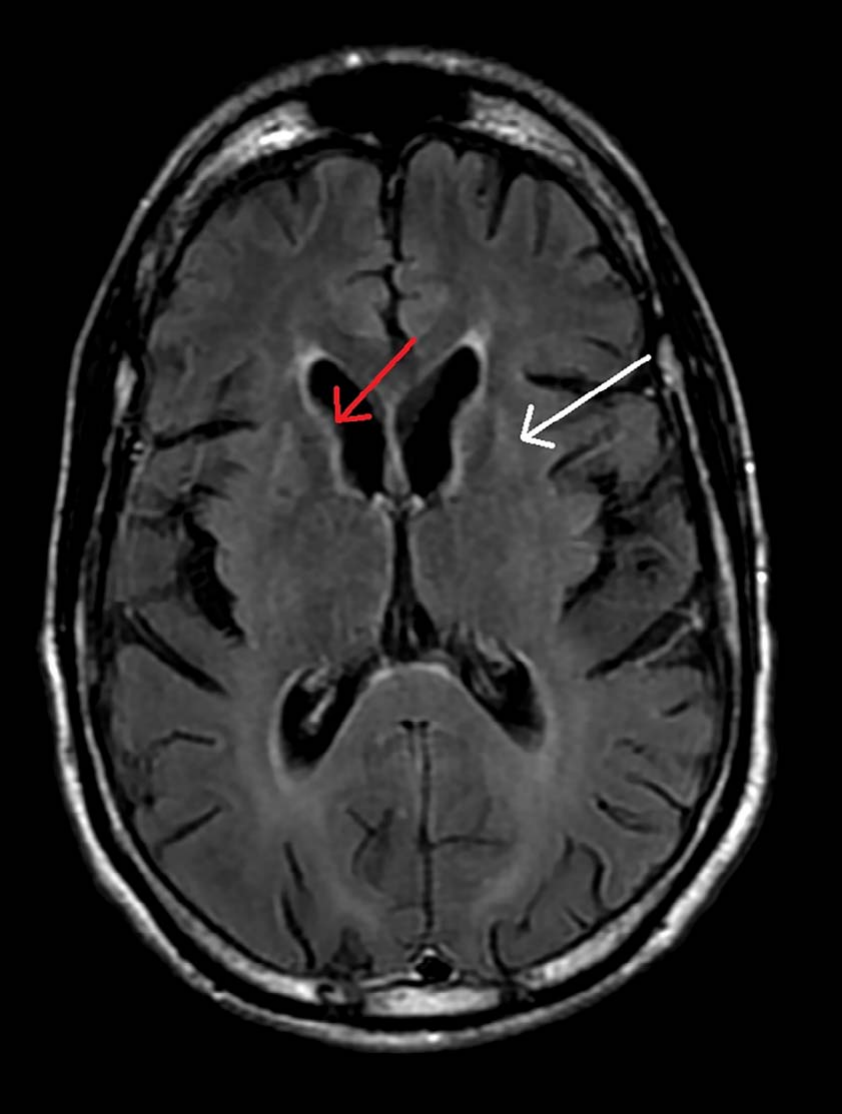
Axial T2-FLAIR (fluid-attenuated inversion recovery) magnetic resonance image of the brain of Case 2 shows severe volume loss in the caudate heads and putamina bilaterally. The right caudate head is indicated with a red arrow. The left putamen is indicated with a white arrow.
